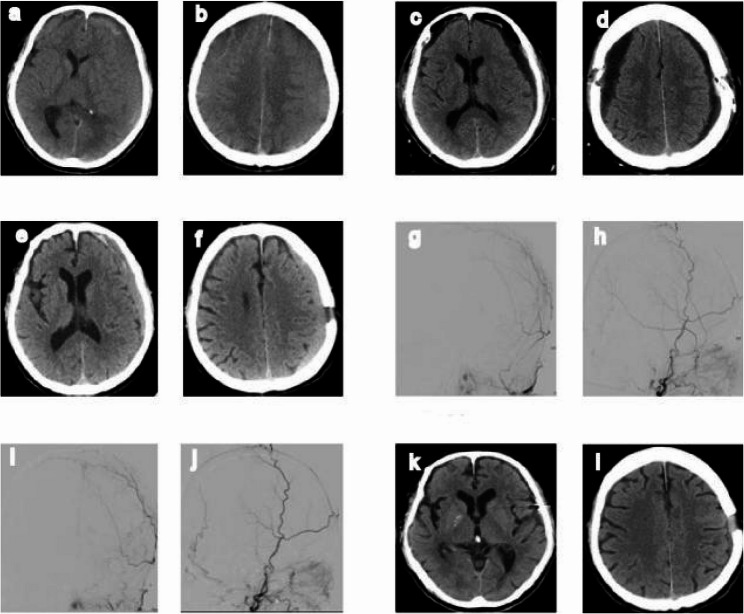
Middle meningeal artery embolization for recurrent chronic subdural hematoma after drilling and drainage. a-f and k-l are computed tomography figures, g-j are digital subtraction angiography figures. a and b indicate bilateral chronic subdural hematoma, c and d are pictures reviewed after drilling and drainage, e and f indicate recurrence of chronic subdural hematoma on the left side. The left middle meningeal artery can be seen in g and h, and i and j suggest that the middle meningeal artery is embolized. k and l are the left chronic subdural hematoma that has been absorbed
